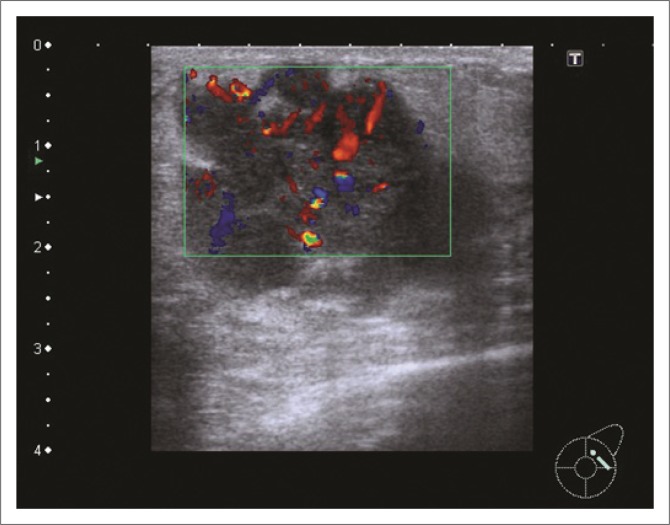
Ultrasound image of the hypoechoic mass in the left breast demonstrating posterior enhancement and increased vascularity.
